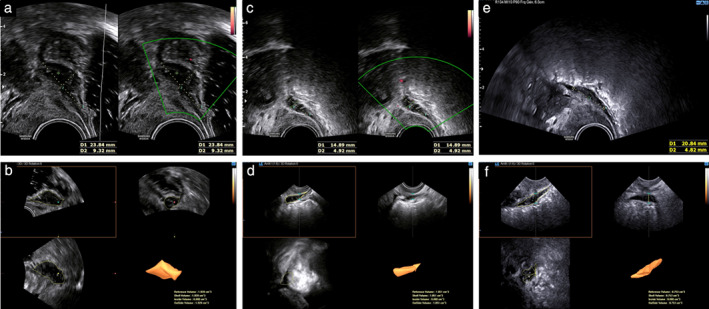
Transvaginal rectosonography, showing endometriotic rectal lesion in two‐dimensional (a,c,e) and three‐dimensional multiplanar (b,d,f) modes before (a,b) and 1 month (c,d) and 3 months (e,f) after transrectal high‐intensity focused ultrasound treatment. Intestinal endometriotic nodule appears as hypoechogenic lesion protruding into intestinal lumen. Volume is calculated using Virtual Organ Computer‐aided AnaLysis (VOCAL) mode (b,d,f). Initial diameters and volume (24 × 9 mm and 1.9 cm^3^) decreased over time (15 × 5 mm and 1.1 cm^3^ at 1 month and 20 × 5 mm and 0.75 cm^3^ at 3 months).
